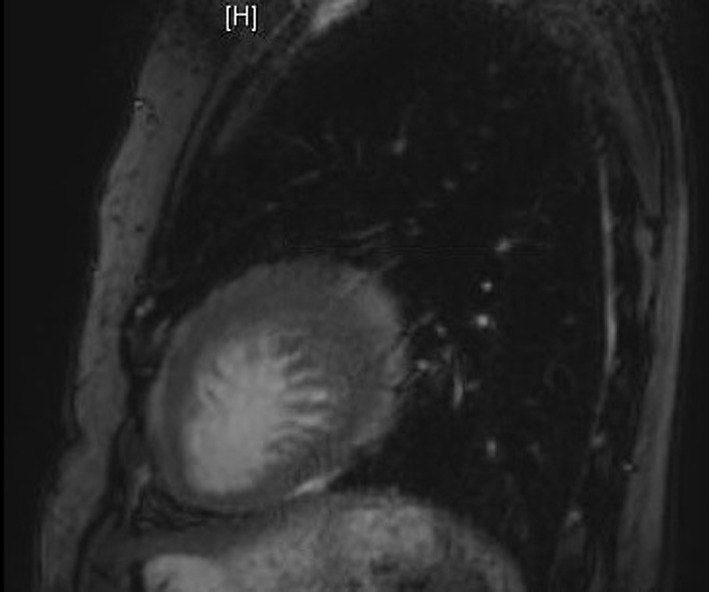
Cardiac MRI shows prominent trabecular processes in the left ventricular cavity.
